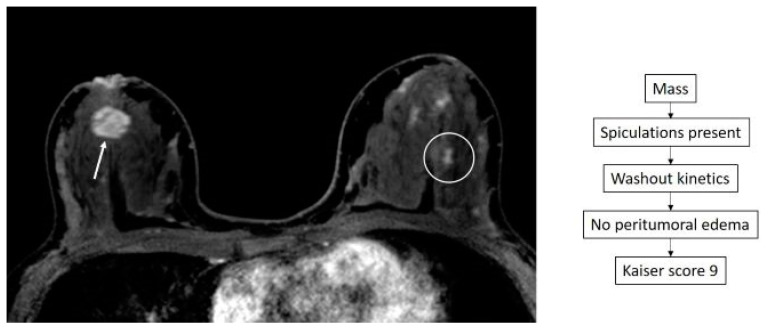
True positive case of a 37-year-old woman with recently diagnosed IDC in the central aspect of the right breast (arrow). An additional small irregular enhancing mass is identified in the lower outer quadrant of the contralateral breast (circle), showing a Kaiser score of 9. MRI-guided biopsy was performed yielding DCIS.
